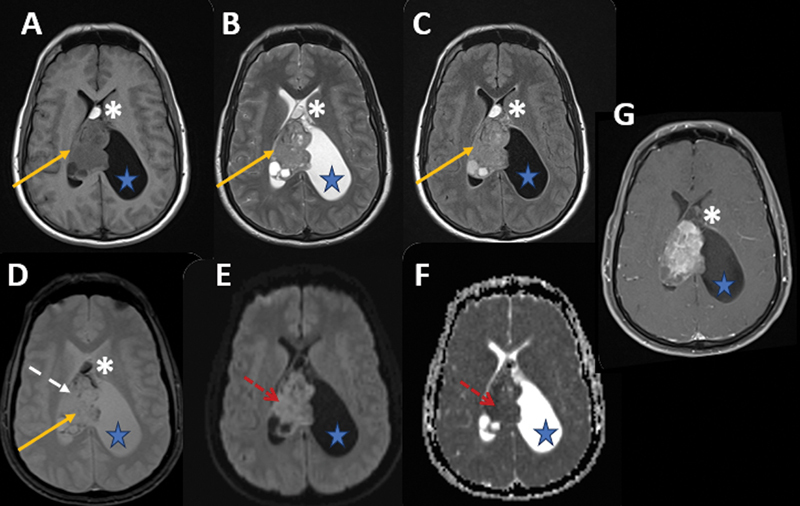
Axial brain magnetic resonance (MR) images on T1-weighted (
